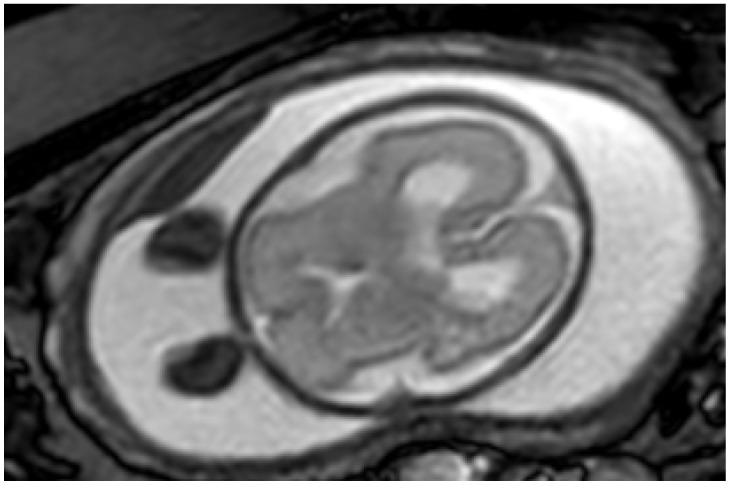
Axial MR image of a fetus at 25 weeks of gestation demonstrating bilateral mild ventriculomegaly.
